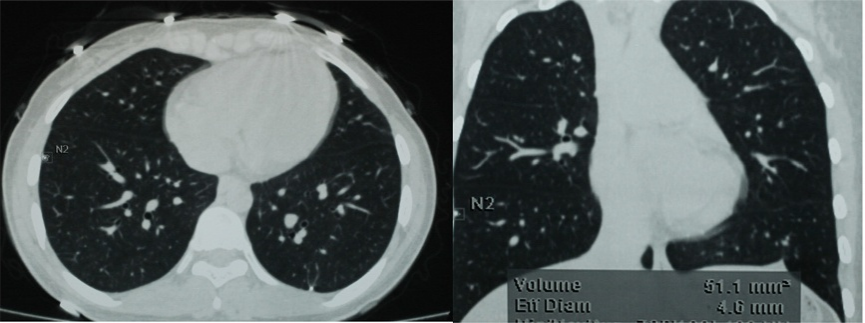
34 months after the first surgery, multiple nodules were found by CT scan in both of her lungs on a follow-up visit.
